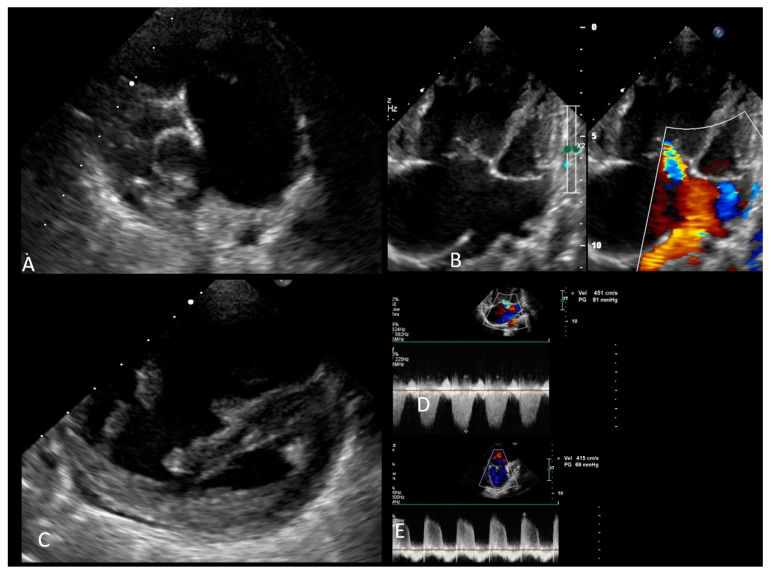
Preoperative echocardiography. (A) Parasternal short axis: the severely dilated PA; (B) 4 chamber view: large ASD, with severely dilated right atrium and right ventricle; (C) short axis view at the ventricles: large right ventricle and the small left ventricle; and (D,E) pulsed tricuspid and pulmonary Doppler highlights elevated pressure in the PA. Legend: ASD—atrial septal defect; PA—pulmonary artery.
